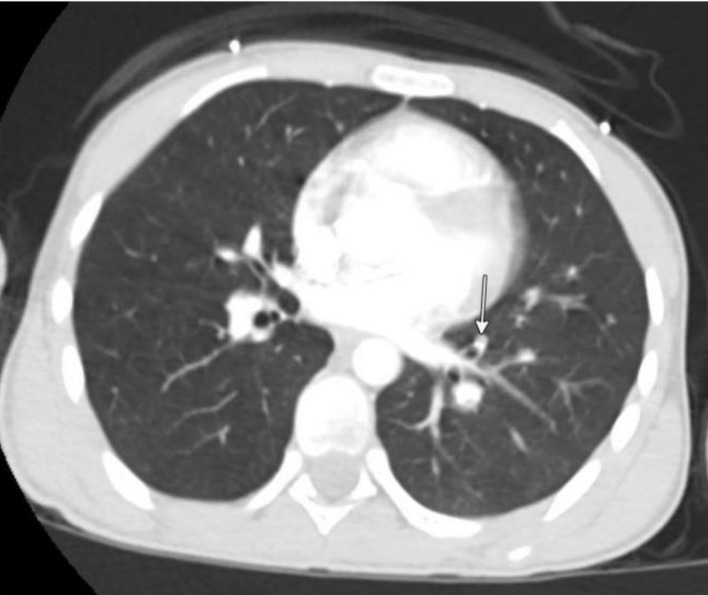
Computed tomography of the chest. Arrow points to one of many pulmonary emboli.
